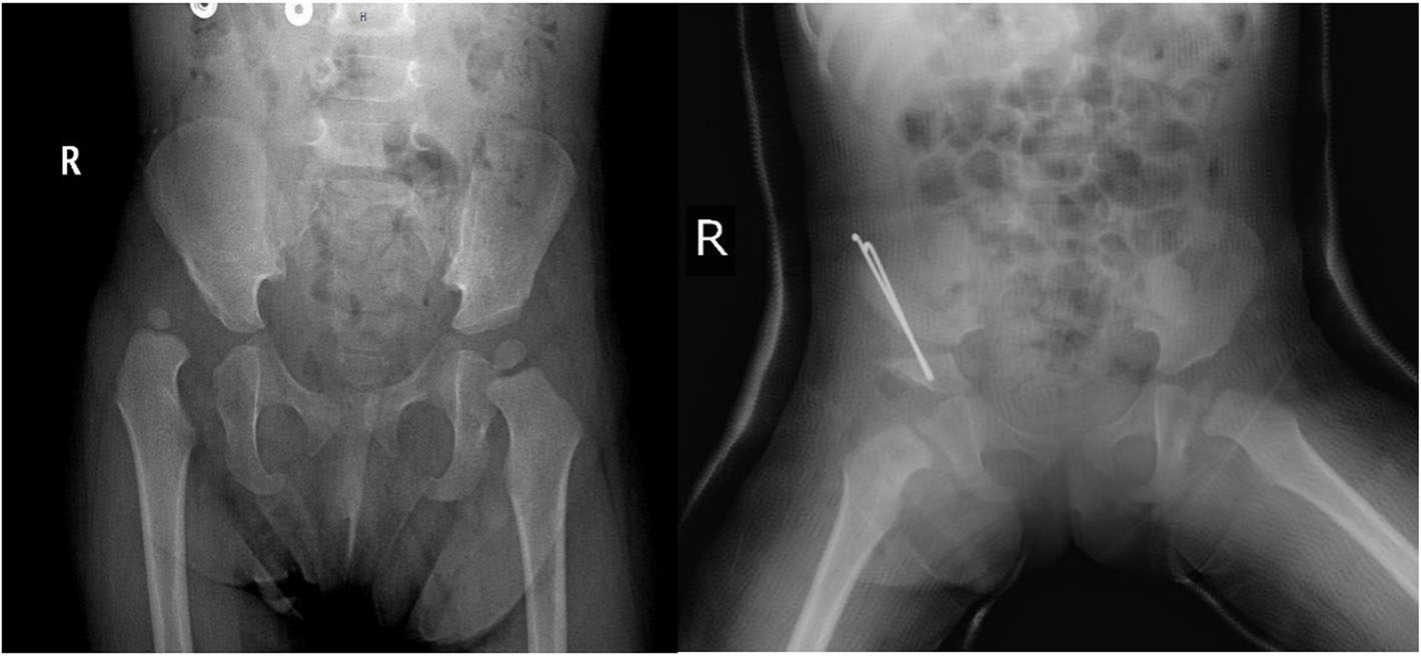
Twenty-one-month-old female, right DDH preoperative and postoperative X-ray images
